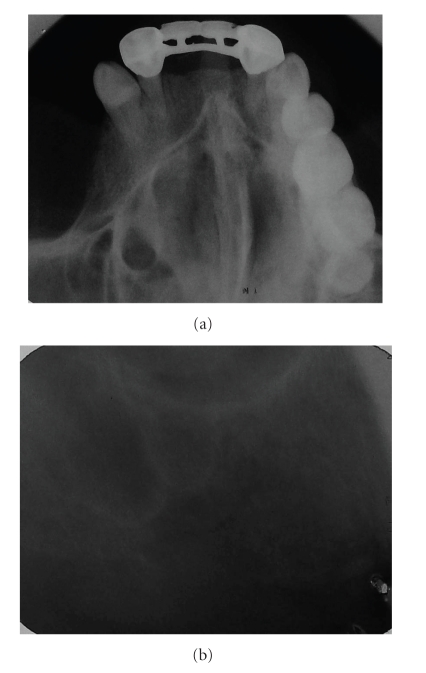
(a) Postoperative maxillary oclusal X-ray. (b) Postoperative periapical X-ray. Notice the complete removal of the right maxillary foreign body.
